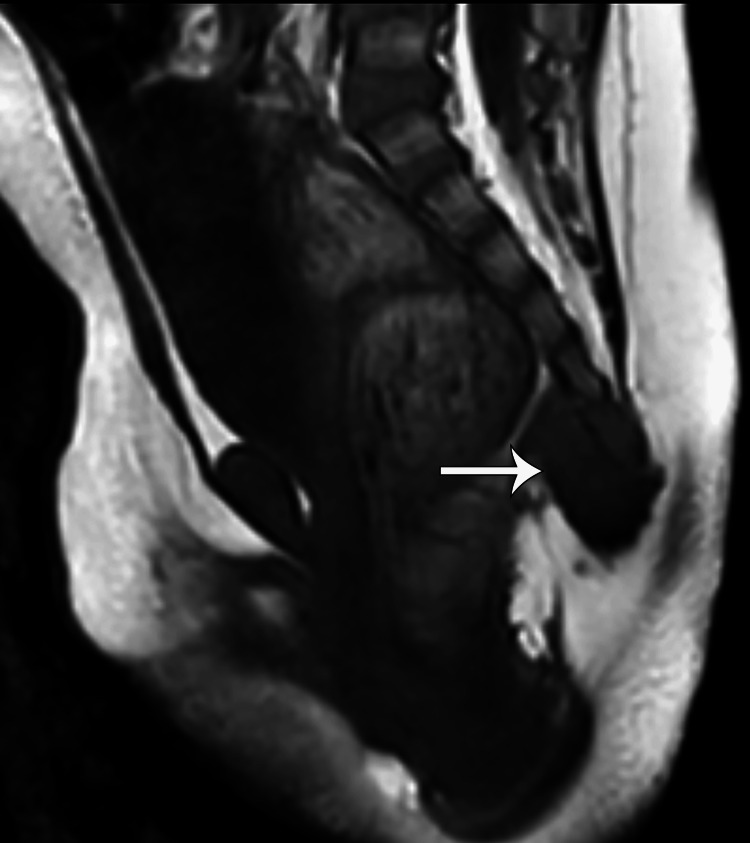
Pre-contrast T1-weighted sagittal MRI image showing a homogeneously hypointense lesion (white arrow) abutting the coccyx. No intrinsic areas of T1 hyperintense to suggest fat, calcification, or hemorrhage. No osseous anomaly or definitive erosion. Clear fat plane between lesion and rectum
